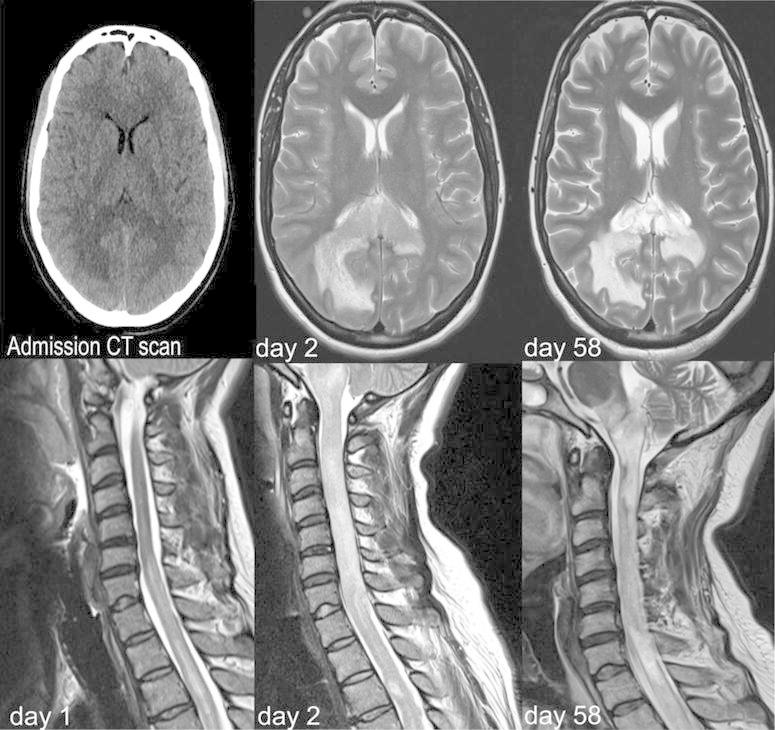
Baseline cerebral CT scan (*upper left side*) and cerebral and spinal MRI at days 1, 2, and 58, respectively
